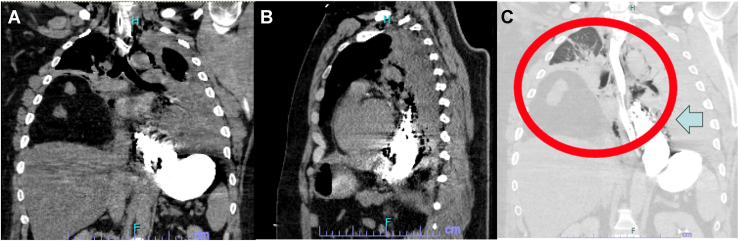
(A, B) Computed tomography showing an esophageal leak and (C) a Morgagni diaphragmatic hernia filling the right hemithorax. The circle and arrow show extravasation of oral contrast material in the distal esophagus and pneumomediastinum.
